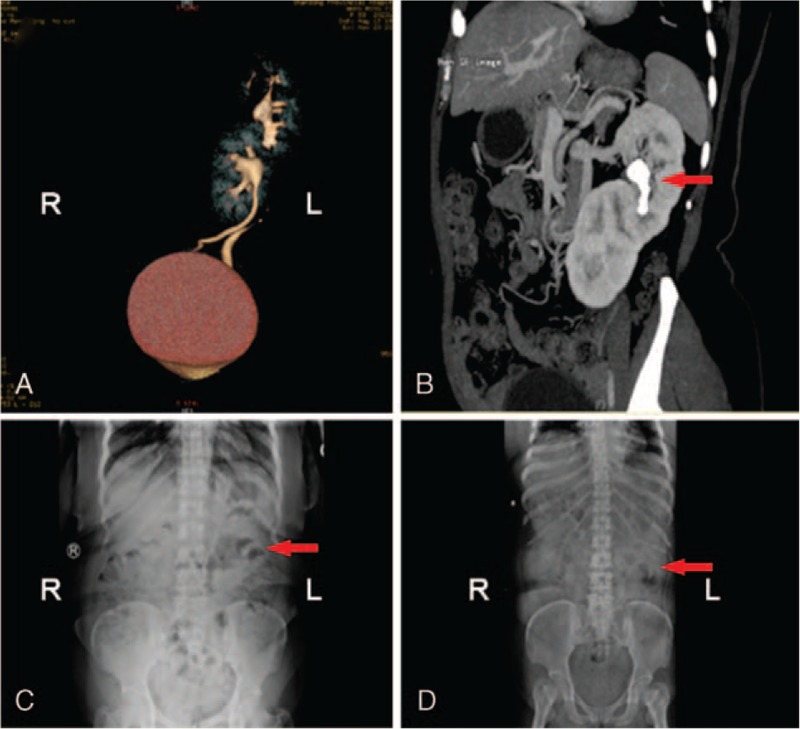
3D computed tomography and X-ray images of patient 1. (A) 3D computed tomography revealed S-shaped right-to-left crossed-fused renal ectopia. (B) CT demonstrated the vascular anomaly and calculi in the left renal pelvis (arrow). (C) Preoperative abdominal X-ray revealed stone shadows (arrow) in the left abdominal area. (D) Postoperative KUB showed no stone shadows.
